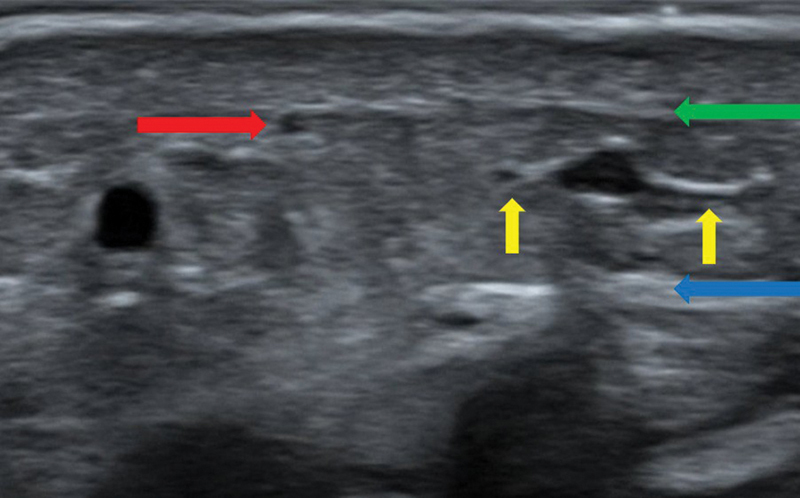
Ultrasonography of lymphatic vessels and veins. A 22 MHz hockey stick probe was used. The red arrow shows the lymphatic vessel. The green arrow shows the superficial fascia. The blue arrow shows the deep fascia. The yellow arrows show two smaller side branches of the vein. Those are a good choice for performing a lymphovenous anastomosis, using the Venturi effect.
